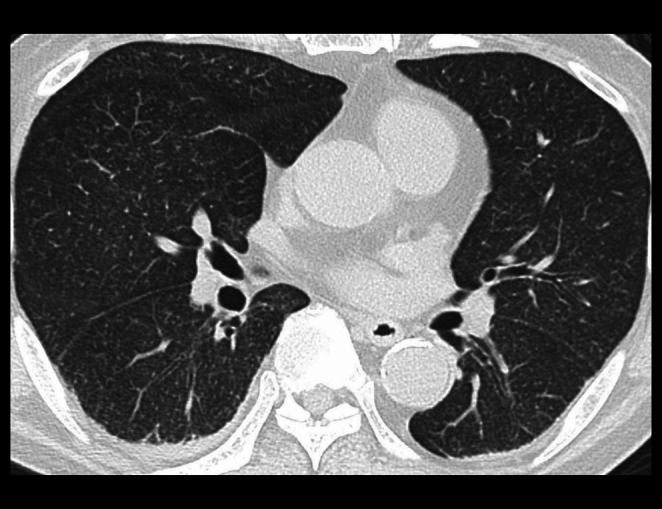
Chest computed tomography (slice thickness: 1 mm) revealed micronodules with a diffuse distribution unrelated to the lobular structure, uniformly distributed throughout the lungs.
